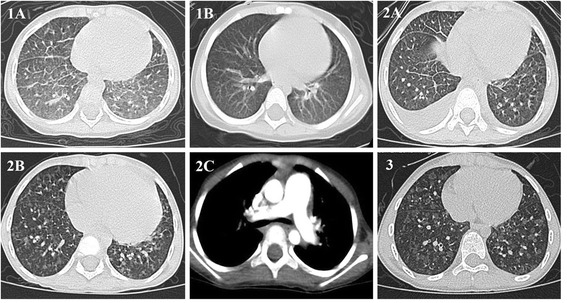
Lung CT showing the presence in both lungs of *1a* diffuse ground-glass opacification predominant in lower region of lung and areas with smooth thickening of interlobular septum (on admission; Patient 1), *1b* absence of abnormal pulmonary feature(after 1 month of treatment; Patient 1), *2a* interlobular septal thickening and bilateral pleural effusion (1 year before admission; Patient 2), *2b* diffuse poorly defined centrilobular nodules(5 days after treatment; Patient 2), *2c* pulmonary artery (PA) with an enlarged diameter exceeding the aorta(5 days after treatment; Patient 2), and *3* diffuse poorly defined ground-glass centrilobular nodules(on admission; Patient 3)
